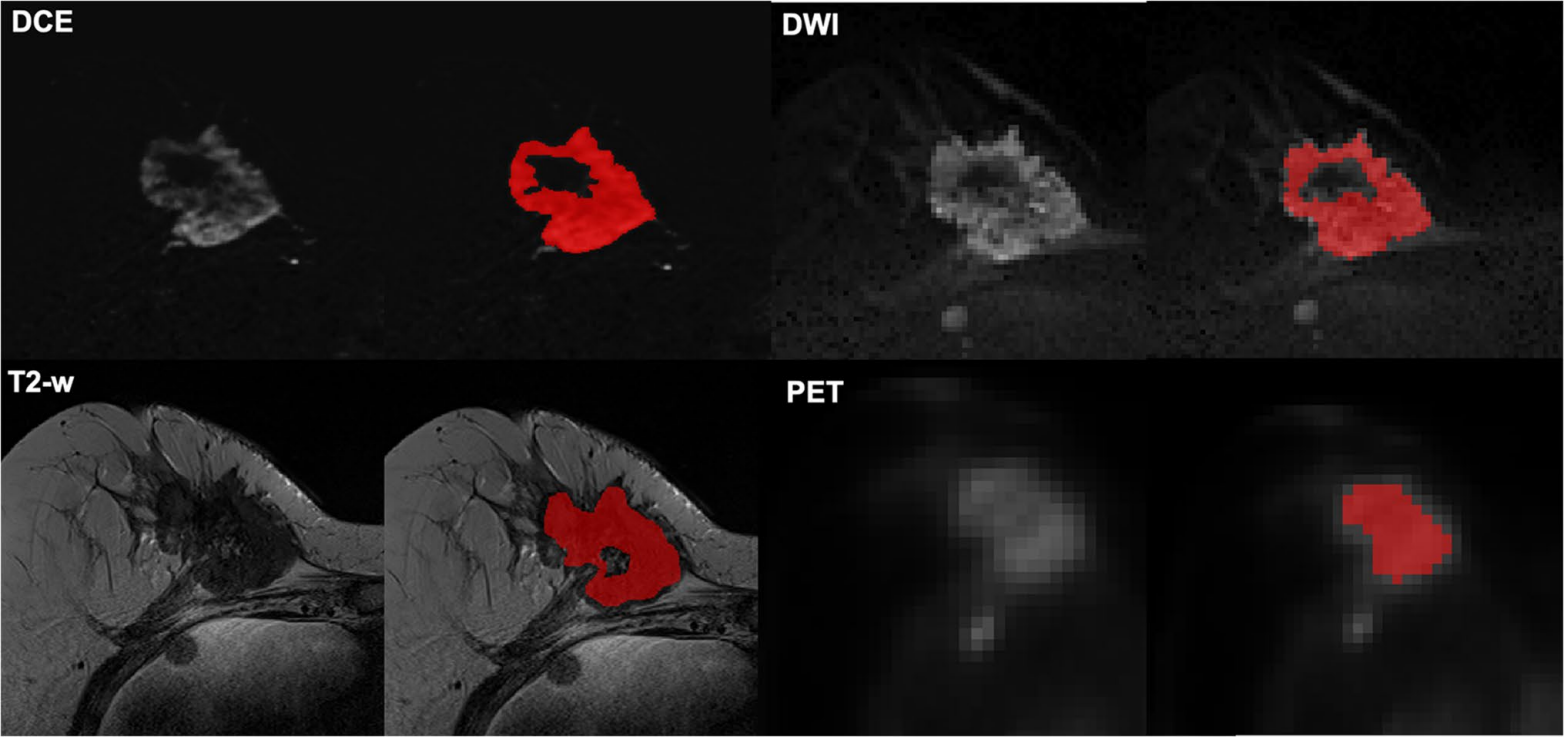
Example of tumor segmentation in a 62-year-old patient with a stage IV invasive ductal carcinoma (G3, luminal B) of the right breast. Three-dimensional regions of interest (ROIs) were drawn over breast lesions on dynamic contrast-enhanced magnetic resonance (DCE-MR) (A), T2-weighted (B), diffusion-weighted (DW) (C), and positron emission tomography (PET) (D) images using a semi-automated method
